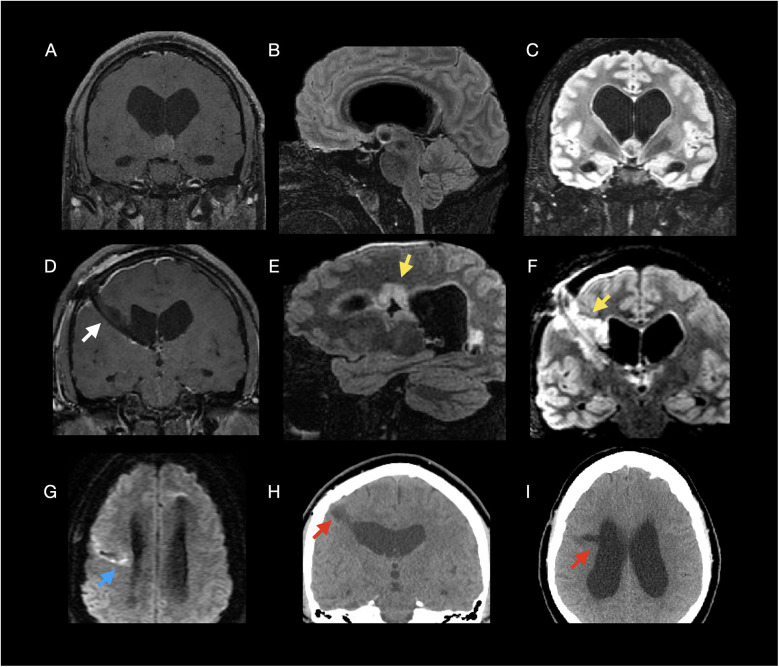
Multimodal imaging of a 24-year-old male with headache due to a symptomatic colloid cyst, treated with endoscopic resection. Preoperative MRI (A–C): Coronal (A) and sagittal (B) post-contrast T1-weighted, and coronal 3D T2-weighted FLAIR (C) images demonstrate a 1.6 cm homogeneously enhancing lesion in the anterior third ventricle at the foramen of Monro, consistent with a colloid cyst, causing obstructive hydrocephalus. Postoperative day 1 MRI (D–G): Coronal post-contrast T1-weighted (D), sagittal (E) and coronal (F) 3D T2-weighted FLAIR, and axial DWI (G) show expected postoperative changes without residual enhancement, with hyperintensity surrounding the resection bed and a hypointense tract along the endoscopic trajectory consistent with postoperative edema and parenchymal injury; DWI (G) demonstrates hyperintensity consistent with diffusion restriction. ThreE–Month folloW,Up CT (H,I): Coronal (H) and axial (I) non-contrast CT images show chronic hypodense parenchymal loss extending toward the right lateral ventricle, consistent with postoperative sequelae. Arrow key: yellow arrows = postoperative hyperintensity (E,F); white arrow = hypointense surgical tract (D); blue arrow = diffusion restriction (G); red arrows = parenchymal loss (H,I).
